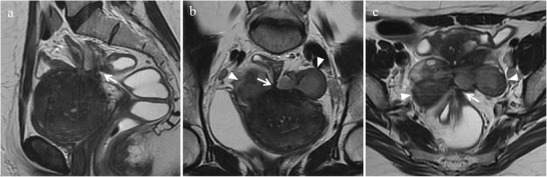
Adhesions in a 40-year-old woman with dysmenorrhea, who underwent two previous surgical interventions for endometriosis. (a) Sagittal, (b) oblique axial, and (c) oblique coronal T2-weighted images show spiculated hypointense strands arranged in confluent angles (white arrows) with loss of the cleavage planes among the anterior surface of the sigma, the posterior uterine serosa and bilateral endometriomas (white arrowheads)
